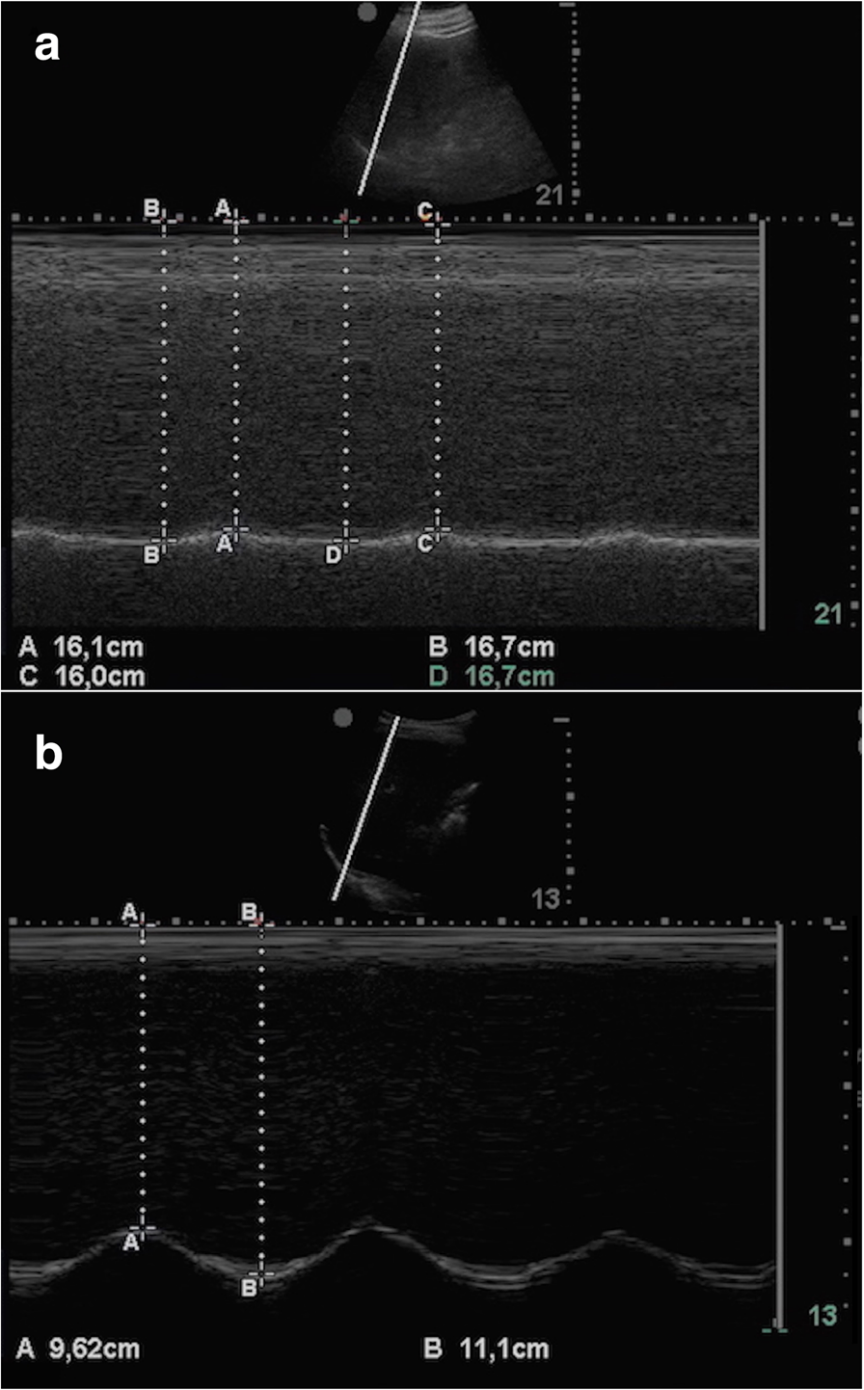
M-mode sonography of the diaphragm of a a representative patient with an impaired right diaphragmatic displacement (DD = 6 mm) and b a representative patient with a conserved right hemidiaphragm function (DD = 14.8 mm). DD was measured on the vertical axis of a frozen image from the baseline, at the end of expiration, to the point of maximum height of inspiration
